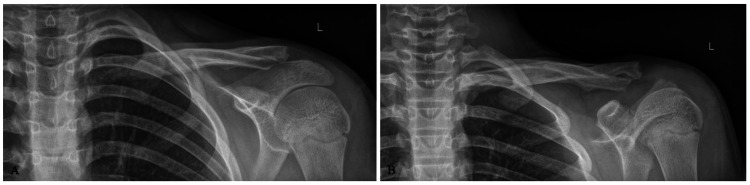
Three-month post-injury radiographs, upright anteroposterior and Zanca views (A-B), demonstrating continued fracture healing and early remodeling.
